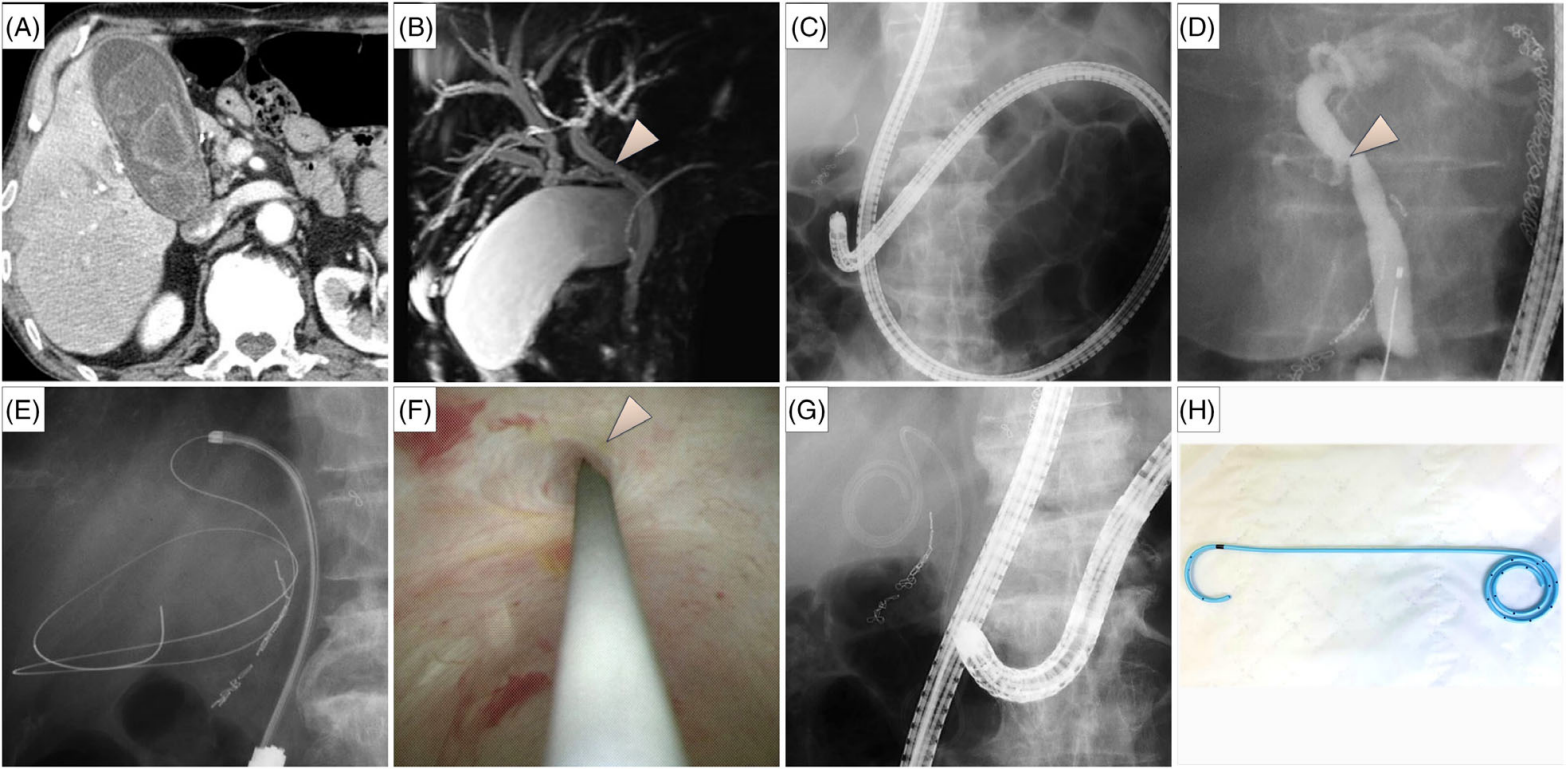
(A) Computed tomography showed findings consistent with acute cholecystitis, including gallbladder distention, wall thickening, and pericholecystic fluid. Multiple gallstones were present. (B) Magnetic resonance cholangiopancreatography revealed an anomalous junction in which the right hepatic duct joined the cystic duct. An arrowhead indicates the confluence of the cystic duct and common bile duct. (C) Endoscopic transpapillary gallbladder drainage was performed using a short‐type single‐balloon enteroscope (SIF‐H290; Olympus Medical Systems, Tokyo, Japan). (D) Cholangiography showed the caudal branching of the cholecystic duct indicative of challenging guidewire insertion into the gallbladder. (E,F) Guidewire access to the gallbladder was successful under cholangioscopic guidance. (G) A 5F plastic stent (IYO‐stent; Gadelius Medical, Tokyo, Japan) was placed into the gallbladder. (H) Specifically designed for transpapillary gallbladder drainage, this 5F stent features a proximal spiral structure with multiple side holes and a distal pigtail shape to prevent migration.
